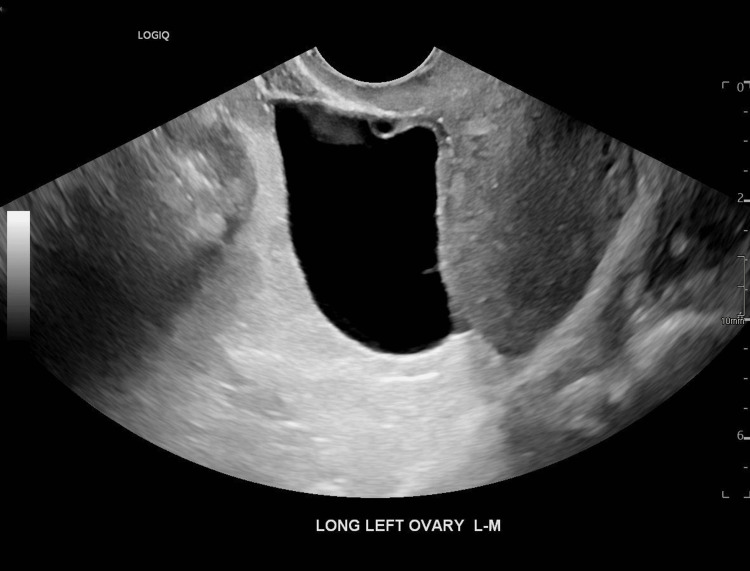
Transvaginal ultrasound demonstrating a 5 cm left ovarian cyst in long-axis view
